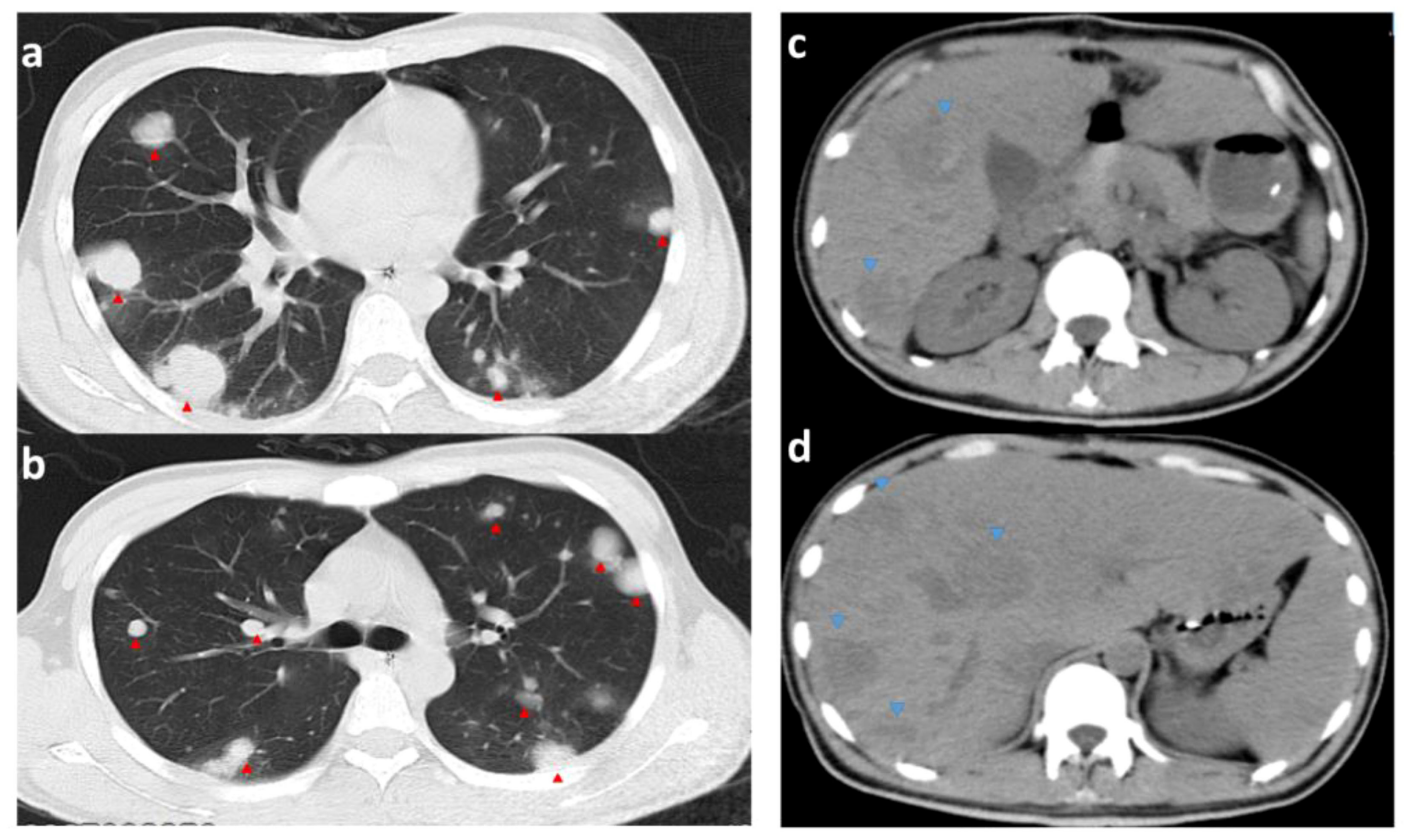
CT images of the chest and abdomen on the 6th postoperative day. (A, B) are CTs of the chest (pulmonary window); (C, D) are CTs of the abdomen; red arrows point to metastases in the lungs (significantly more extensive and numerous than before surgery). Blue arrows point to liver metastases (hypointense shadow significantly larger than before surgery).
